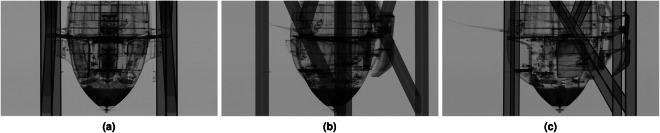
Three X-ray projections of the front part of the airplane fuselage which were captured at different rotation angles: 0° (see a), 45° (see b), and 90° (see c). The vertical dark structures on the outer area of the images represent the mounting brackets for the CT scan. In the centre of the images, the lower part shows the propeller-like generator, and in the middle section, the pilot’s seat along with the dashboard is visible.
